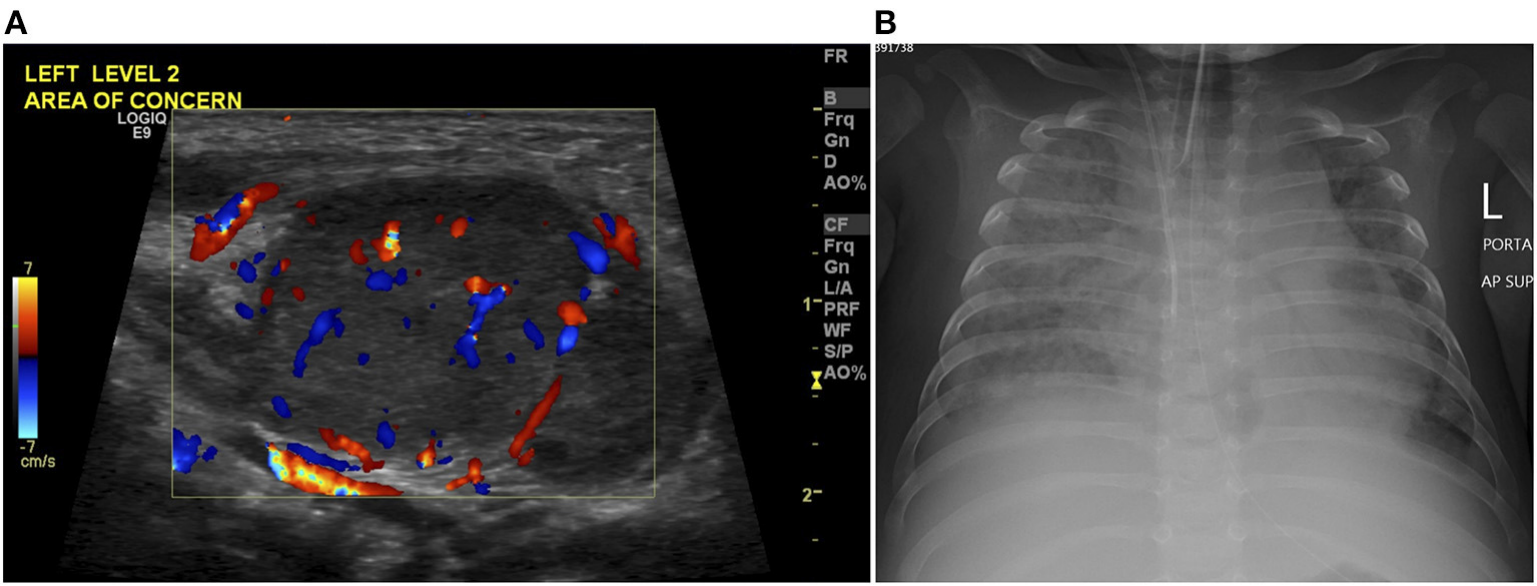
(A) Color Doppler images on neck ultrasound scan at age of 45 days demonstrates an enlarged left cervical lymph node of increased vascularity in keeping with lymphadenitis. (B) Chest radiograph at age of 145 days shows tube and line in position, and bilateral diffuse alveolar-interstitial opacification with right pleural effusion in keeping with pulmonary edema.
